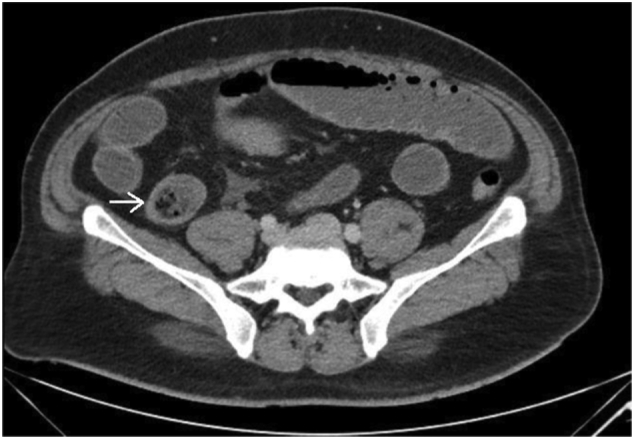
A axial plane of CT abdomen showing an intraluminal mass in the ileum, pointed by the white arrow.
